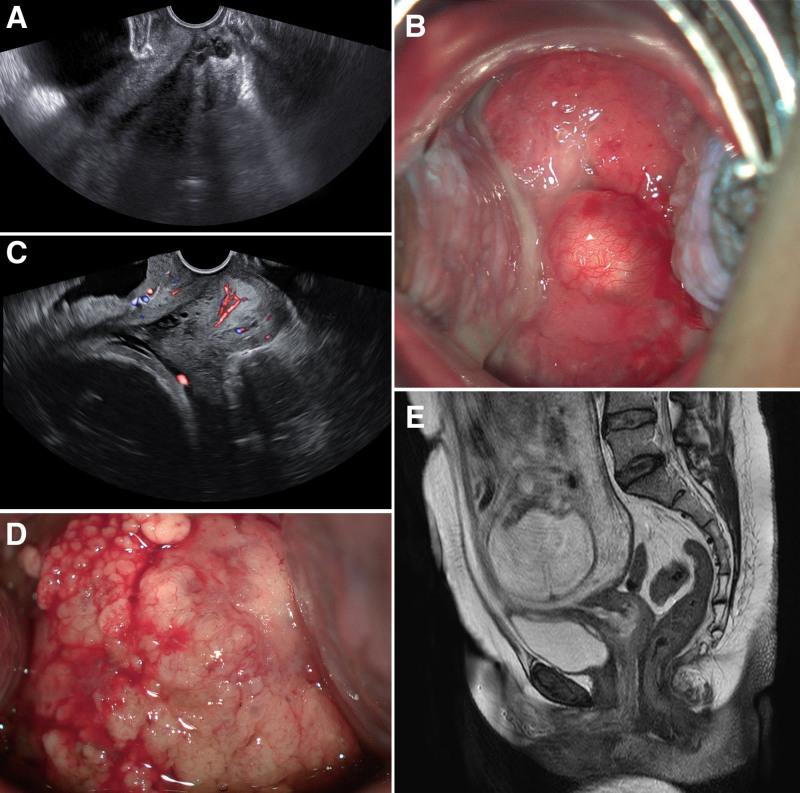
Imaging findings of the patient at 7 weeks and 26 weeks of gestation. (A) Pelvic ultrasound: a cystic lesion around 1.4 × 1.7 cm was seen in the posterior lip of cervix. (B) Colposcopy: a mass with a size of about 1 cm was seen at 6 O’ clock, mimicking a nabothian cyst at 7 weeks of gestation. (C) Colposcopy: 1.5 × 1.5 cm sized cervical mass with papillary nodules after rupture from cystic wall at posterior lip of cervix. (D) Pelvic ultrasound: 1.5 × 1.5 cm sized mass with plentiful vascularization was seen in the posterior lip of cervix. (E) Pelvic MRI without contrast: sagittal T2WI show 1.7 cm sized papillary mass with irregular margin of low signal intensity in the exocervix, at 26 weeks of gestation. MRI = magnetic resonance imaging.
